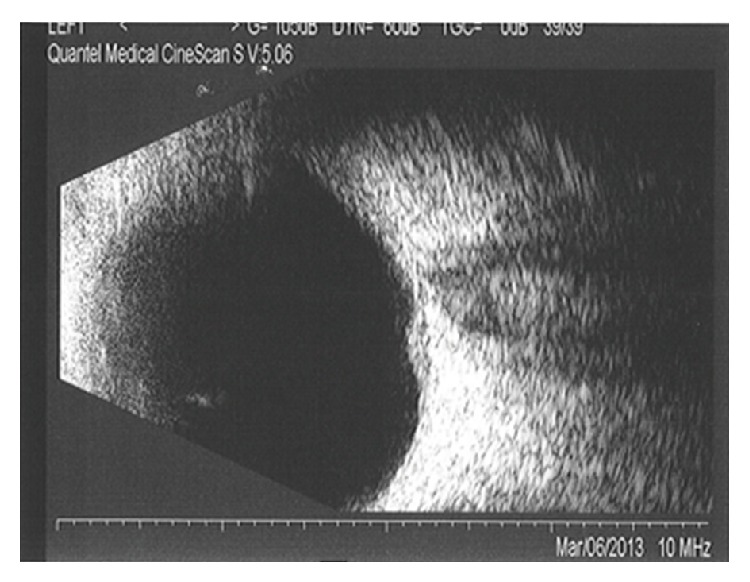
At diagnosis, B-scan ultrasound showed an elevated choroidal lesion with moderate internal reflectivity in the left eye.
